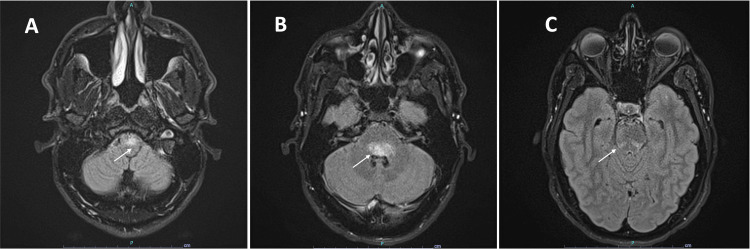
Repeat brain MRI on Day 4 of methylprednisolone treatment Repeat brain MRI on Day 4 of methylprednisolone treatment showing hyperintensities in the anterior medulla, dorsal pons, and dorsal midbrain in the fluid attenuated inversion recovery (FLAIR) sequence compared to MRI at initial presentation.
